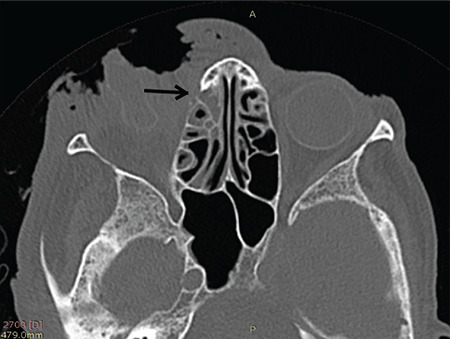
Computed tomography image showing bony erosion of the lamina papyracea of the right orbit caused by *Chrysomya bezziana* larvae
